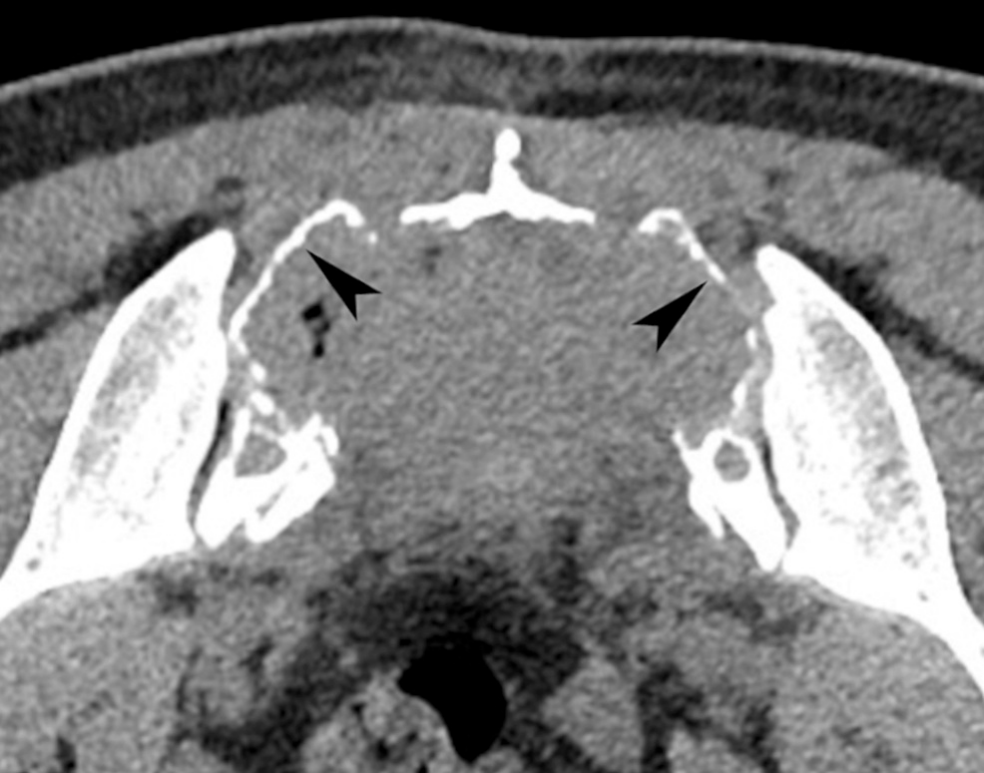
Axial computed tomography section through the first sacral segment. This reveals a lytic, expansile mass with soft-tissue attenuation, spanning the sacral body and alae bilaterally, with thinning but no sclerosis of the cortical bone (arrowheads).
